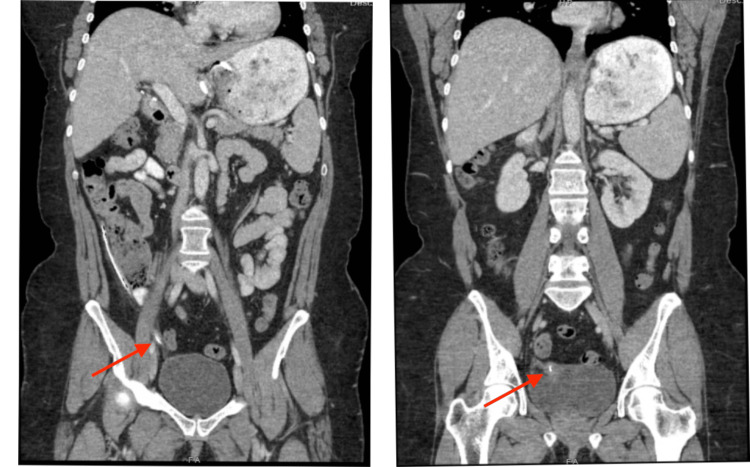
CT imaging revealing the course of the tube in the abdomen (left) and the tubing embedded in the vaginal cuff (right) on sequential coronal sections Red arrows point to the gastric band tubing.
